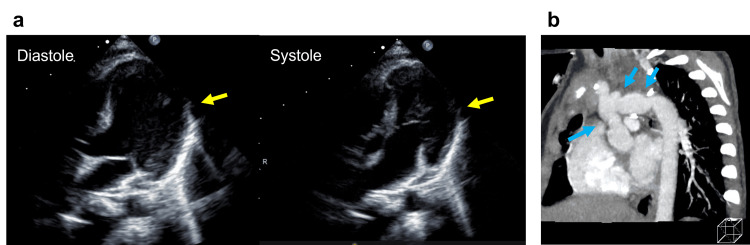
Left ventricular motion and shape of the aortic arch a. The left ventricular long axis view in diastole (left) and systole (right) is shown. The ventricular motion is satisfactory, and no apparent focal dyskinesis is observed. Mild left ventricular hypertrophy is identified (yellow solid arrows). b. The computed tomography angiogram of the aortic arch is shown. While the aortic arch has multiple stenotic lesions (blue solid arrows), the pressure gradient of non-invasive pressure measurements between upper and lower extremities is less than 10 mmHg.
